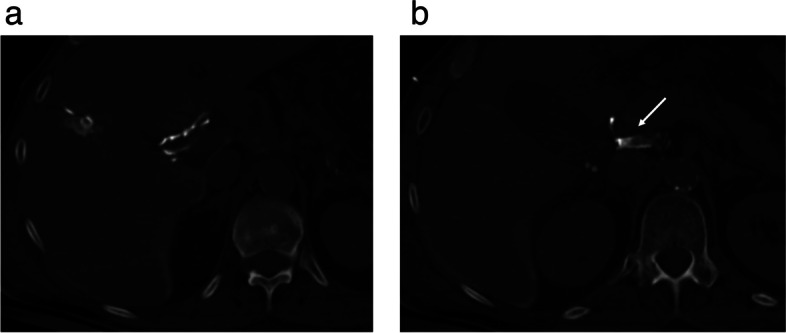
a-c Transhepatic Computed Tomography lymphangiography (a) showing a lymphatic leak (arrow) (b) and subsequent embolization using Glue (1:3) (c)
